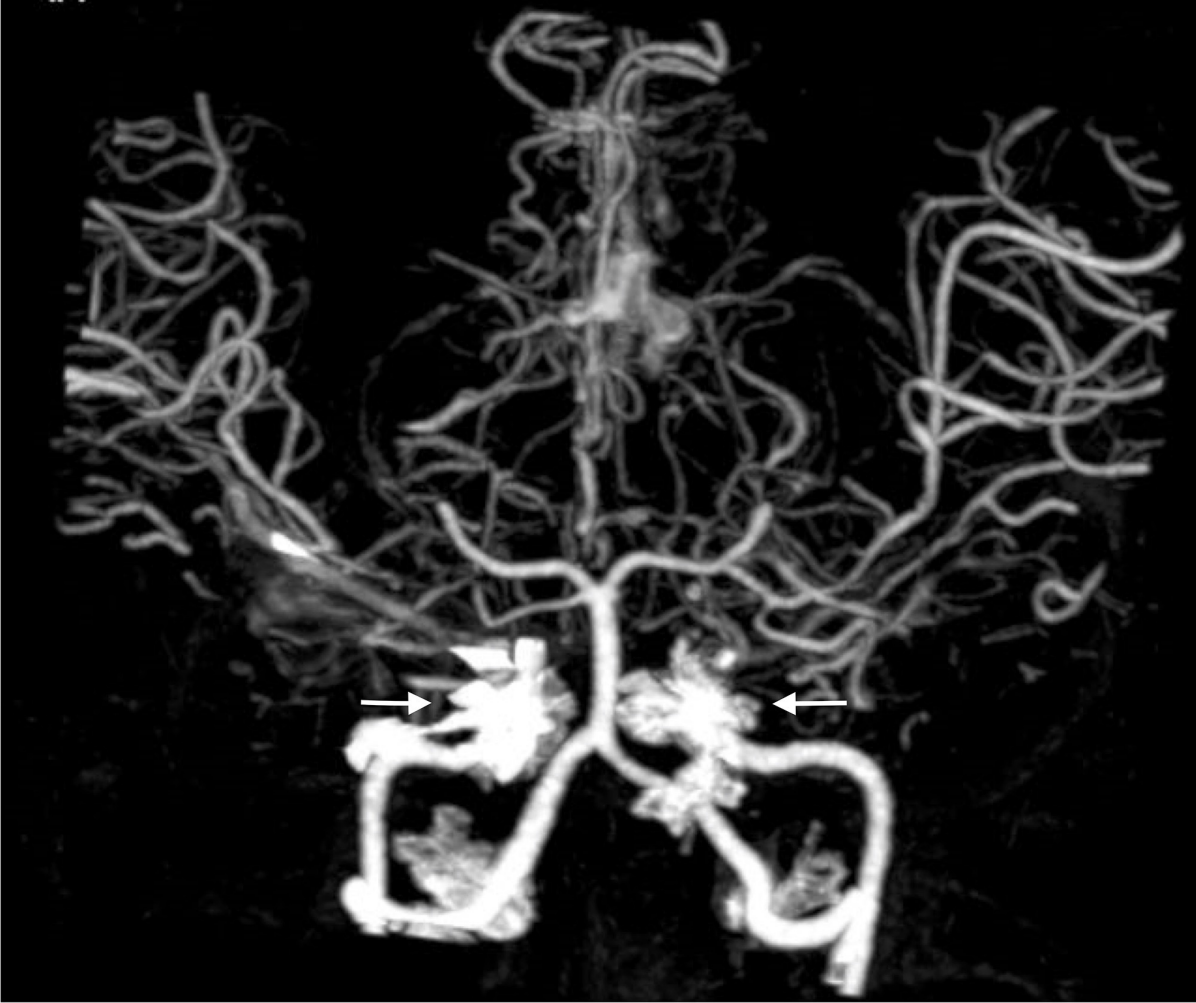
Computerized tomographic angiography (CTA) of the head and neck with contrast demonstrating occlusion of the bilateral supraclinoid internal carotid arteries
